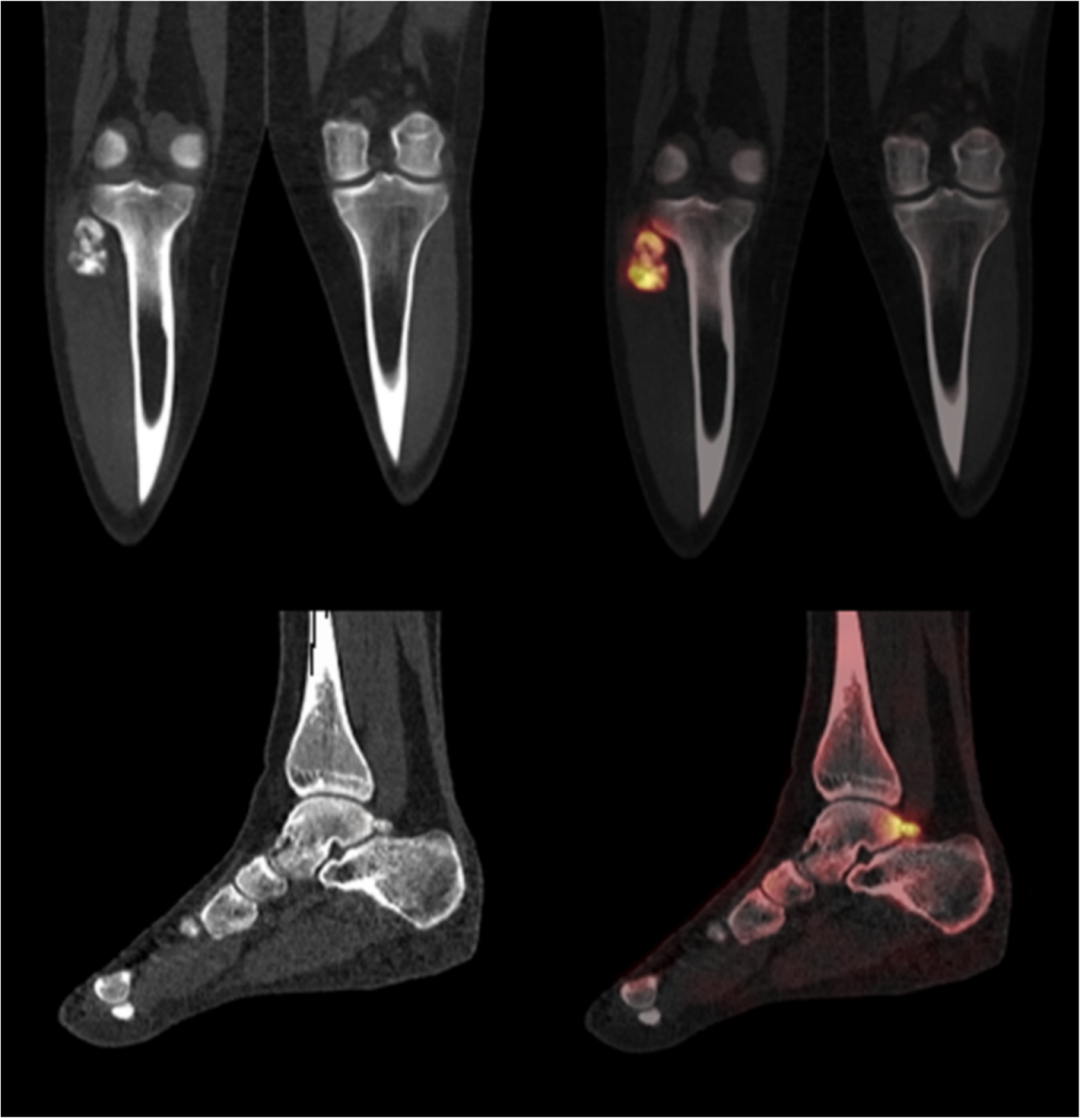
Images of an 18-year-old male’s right leg produced with xSPECT Quant™ and xSPECT Bone™ (Siemens Healthineers), containing a giant cell tumour in the proximal fibula. Uptake in proximal tibio-fibular joint was concluded as normal as it was mild and reactive and no erosion could be seen. Siemens claim that xSPECT Bone™ offers improved image quality and lesion localisation through sharper edge delineation. But is there enough evidence to assume it can be routinely called upon to perform accurate and reproducible quantification to support important clinical decisions? (Images courtesy of Siemens Healthineers, Erlangen, Germany)
